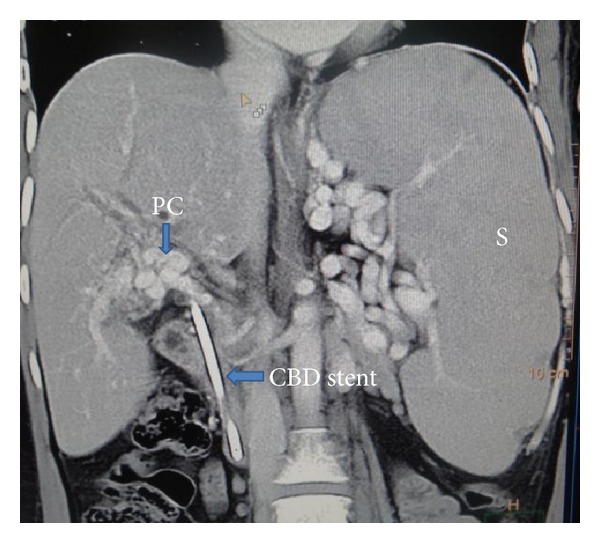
CT arterial portogram showing portal vein replaced by multiple collaterals, that is, cavernomatous transformation of the portal vein also known as a Portal Cavernoma (PC), grossly enlarged spleen (S), and CBD stent in situ for portal biliopathy in a patient with EHPVO.
